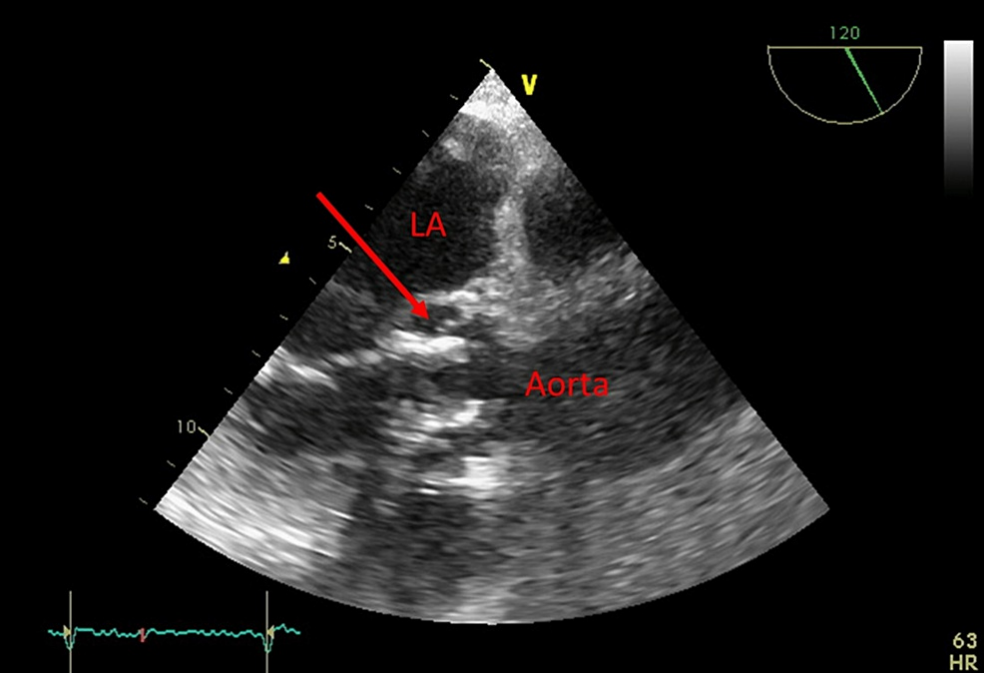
Apical long-axis view on trans-esophageal echocardiogram (TEE) showing a liquefactive abscess (red arrow) near the mitral trigone. LA: left atria; TEE: trans-esophageal echocardiogram
